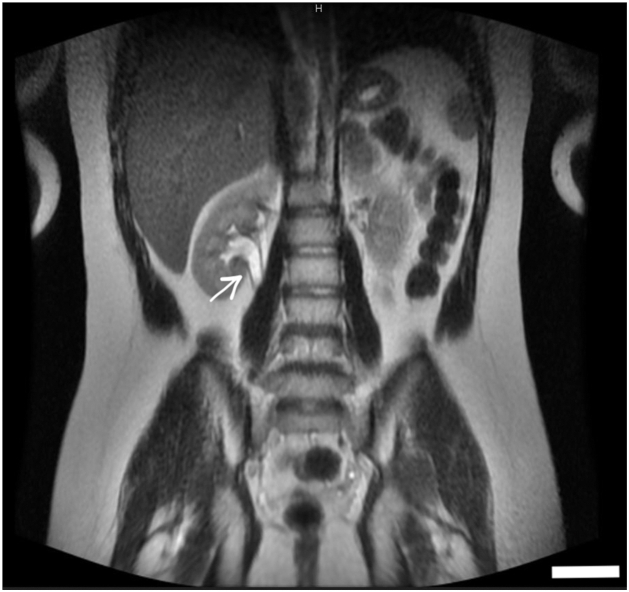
Coronal T2-weighted MRI demonstrating a duplicated *Right* ureter and double collecting system (arrow) with mild dilatation of the *Lower* moiety. The kidneys are in normal anatomical position and size with no evidence of mass or nephrolithiasis.
